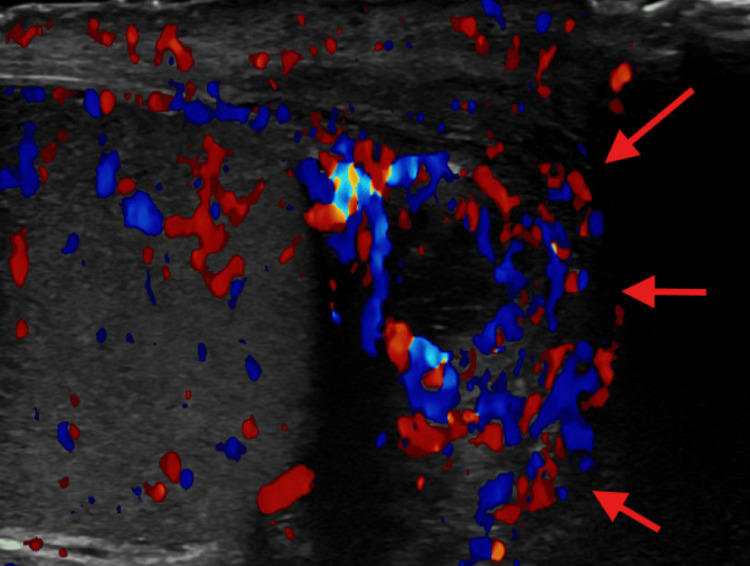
Doppler ultrasound in case 3 of right testicle and epididymal tail which is thickened with mild hyperemia (red arrows).
